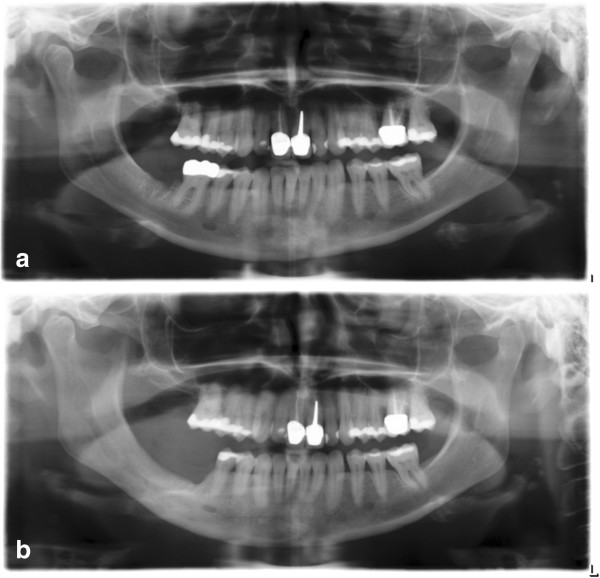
Pre- and postoperative panoramic radiographs. Preoperative radiograph (a) is showing a persistent extraction socket and hypersclerosis of the mandibular bone in the region 46/47 after extraction of 47 in January 2013. Postoperative panoramic radiograph (b) revealing a reduction of the mandibular bone height after repeated osteotomy and adequate ossification of the former extraction sockets.
